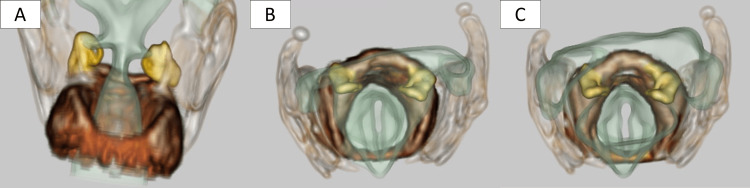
Laryngeal three-dimensional CT scan Arytenoid cartilage does not show any deviation suggestive of dislocation. There is almost no change in the position of the vocal cords during either the inspiration or the vocalization phase, indicating that their movement is highly impaired. (A, B) Inspiration phase. (C) Vocalization phase. Yellow: arytenoid cartilage; dark brown: cricoid cartilage; light brown: thyroid cartilage; light green, V-shaped structure at ventral side: vocal cord.
